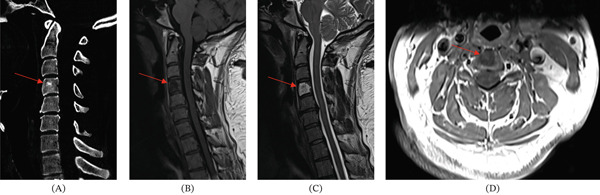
These images of the cervical spine are from another patient treated at our medical center with a likely BNCT diagnosis, showing typical BNCT radiological features for comparison. (A) Panel A shows an intraosseous sclerotic lesion (red arrow) on sagittal CT. (B) Panel B shows low signal on nonenhanced T1 MRI (red arrow). (C) Panel C shows T2 hyperintensity (red arrow). (D) Panel D shows no enhancement on postcontrast axial T1 (red arrow).
